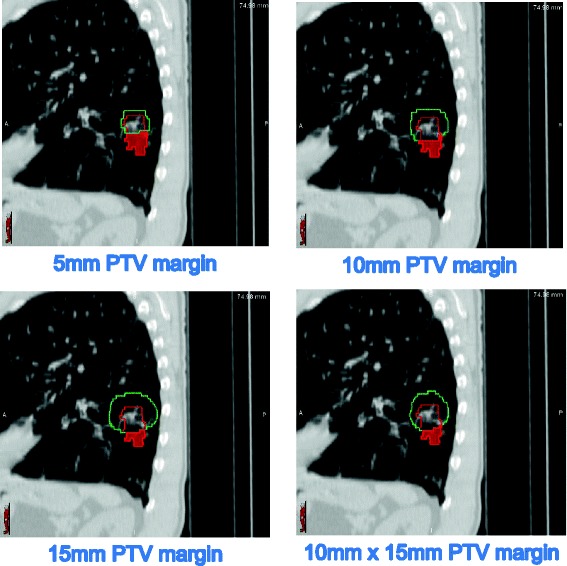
CT scan of a patient with a small lower lobe tumour. The 4D-GTV is shown in red and the 3D-PTV is shown in green. The highlighted red area is the part of the 4D-GTV that was not covered by the 3D-PTV for the margin applied.
